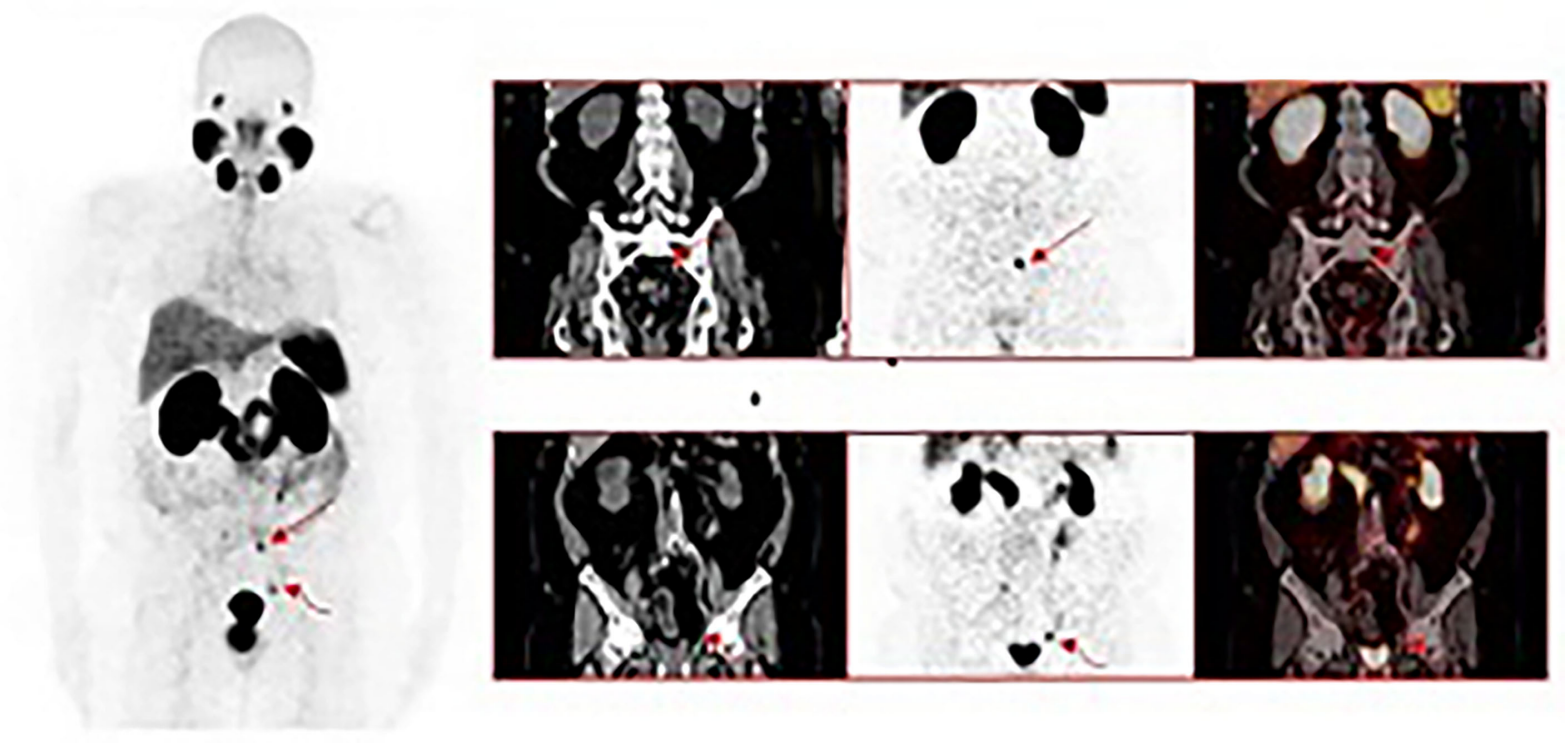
A 63-year-old male with a history of radical prostatectomy for adenocarcinoma of the prostate, Gleason 4 + 4. ^68^Ga-PSMA-11 PET/CT was requested for localization of disease recurrence at a serum PSA of 0.54 ng/mL. Images show intense tracer uptake in a subcentimeter left presacral node (straight arrows) and a subcentimeter left external iliac node consistent with the sites of prostate cancer recurrence. This research was originally published in JCM.Lawal IO, Lengana T, Popoola GO, Orunmuyi AT, Kgatle MM, Mokoala KMG, Sathekge MM. Pattern of Prostate Cancer Recurrence Assessed by 68Ga-PSMA-11 PET/CT in Men Treated with Primary Local Therapy. J Clin Med. 2021 Aug 29;10(17):3883.
